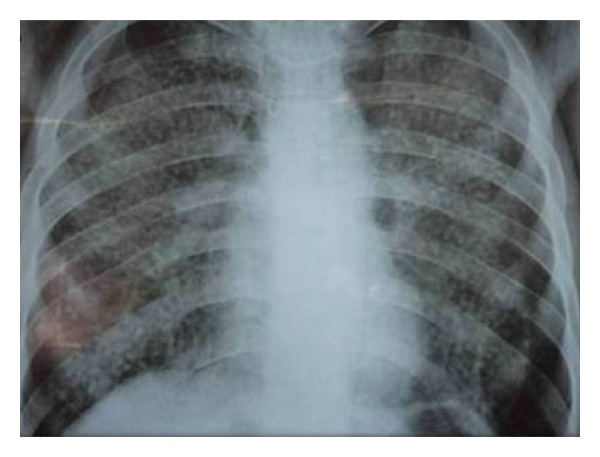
Chest X-ray: bilateral diffuse nodular opacities with bilateral pneumothorax.
